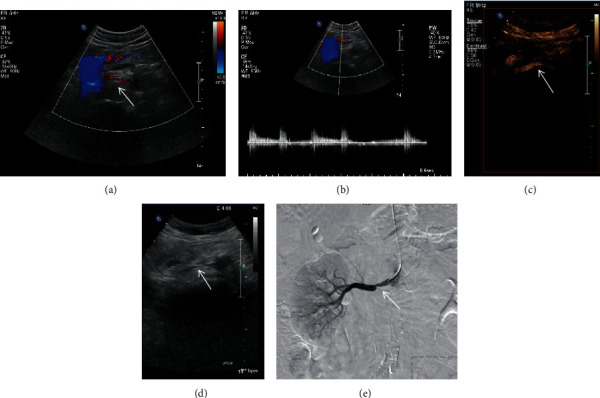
DUS images, CEUS images, and their corresponding DSA images of grade II (stenosis rate: 50%-69%). (a) Color Doppler ultrasound was used to show the long axis section of renal artery. (b) The peak systolic velocity at the stenosed segment of the renal artery was obtained by the pulse Doppler ultrasound. (c) Contrast-enhanced ultrasound can increase the visualization of renal artery and can be used for the display of grade II renal artery stenosis. (d) Two-dimensional grayscale ultrasound cannot clearly show renal artery stenosis. (e) Digital subtraction angiography confirms the result of contrast-enhanced ultrasound and shows the diameter of the main renal artery decreased at the stenosed segment in the patient with renal artery stenosis.
